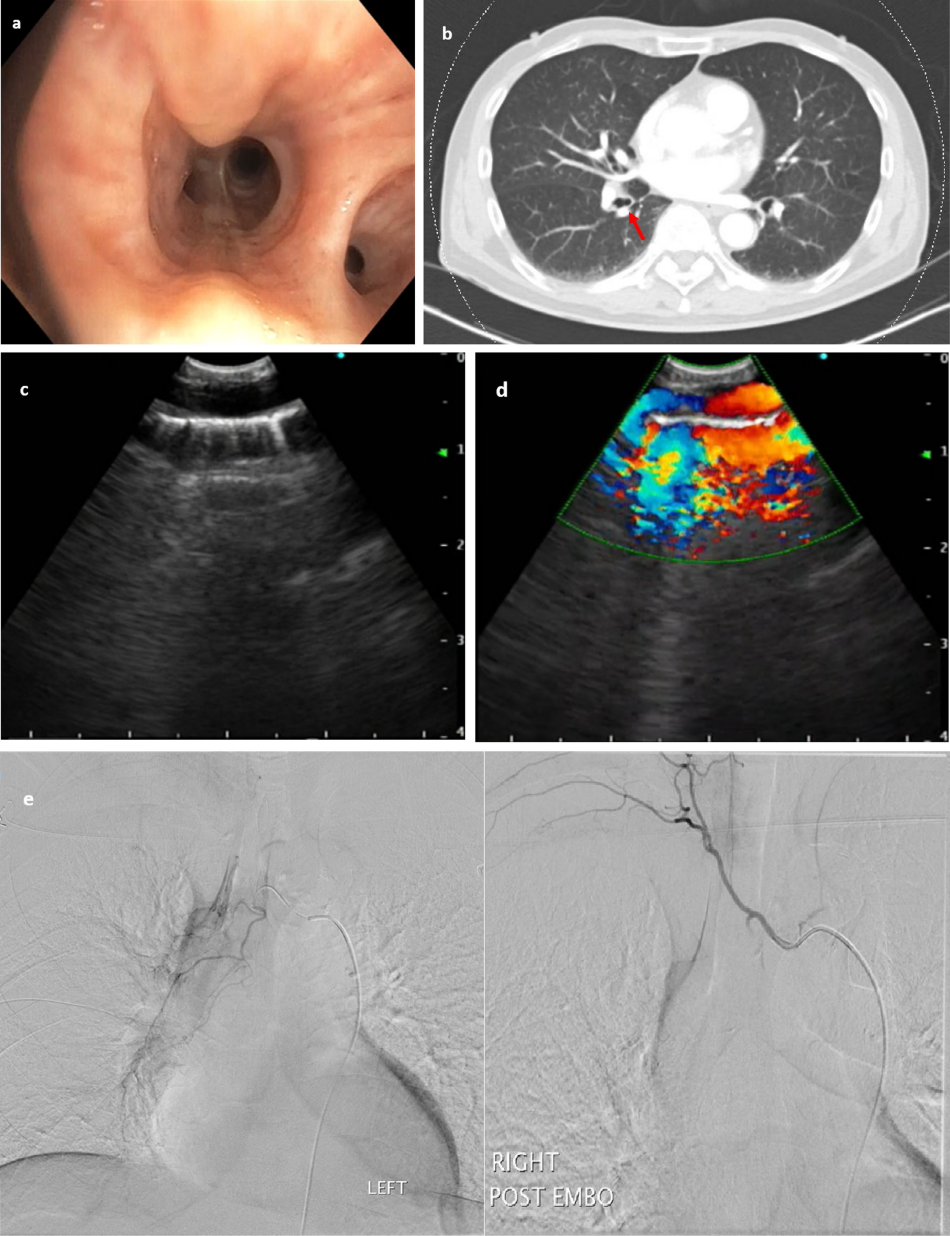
(a) Non‐pulsatile tubular‐like endobronchial lesion at the right lower lobe anterior segment. (b) CT thoracic angiogram with corresponding site of endobronchial lesion seen on bronchoscopy (red arrow) (c) Convex endobronchial ultrasound findings of a linear anechoic structure resembling a vessel. (d) Convex endobronchial ultrasound in colour doppler mode demonstrating blood flow within the linear anechoic structure. (e) Pre‐ and post‐bronchial embolisation images showing successful embolisation of right lower lobe bronchial arteries.
